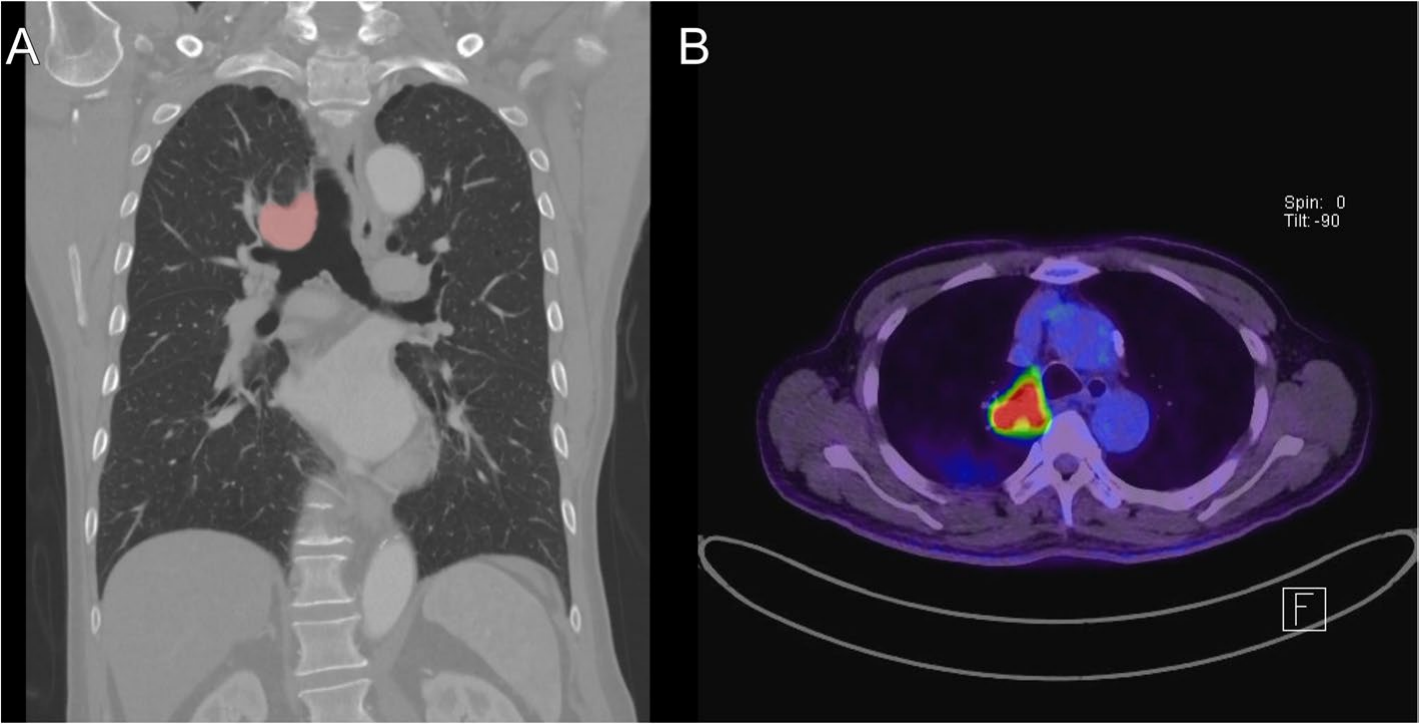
A Contrast-enhanced chest CT scan shows an approximately 4 cm mass obstructing the right upper lobe bronchus orifice. B PET-CT images reveal a central tumor with increased FDG uptake abutting the right lateral wall of the distal trachea. CT, computed tomography; PET-CT, positron emission tomography-computed tomography
